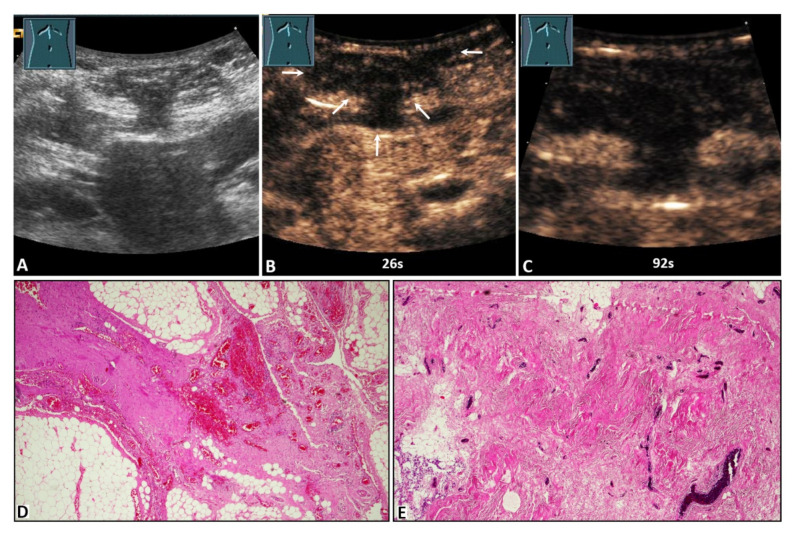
A 59-year-old male patient with acute peritoneal pain in the umbilical region and a palpable tumor. (A) B-mode ultrasound shows a complex lesion located in the abdominal wall, with visualization of omental hernia content. (B,C) On contrast-enhanced ultrasound, the lesion shows absent enhancement after 26 s (arrows) and 92 s. (D) Omentum with fibrotic strands, including dilated hyperemic capillaries (4× magnification). (E) Fibrotic hernia sac with dilated hyperemic capillaries (10× magnification).
